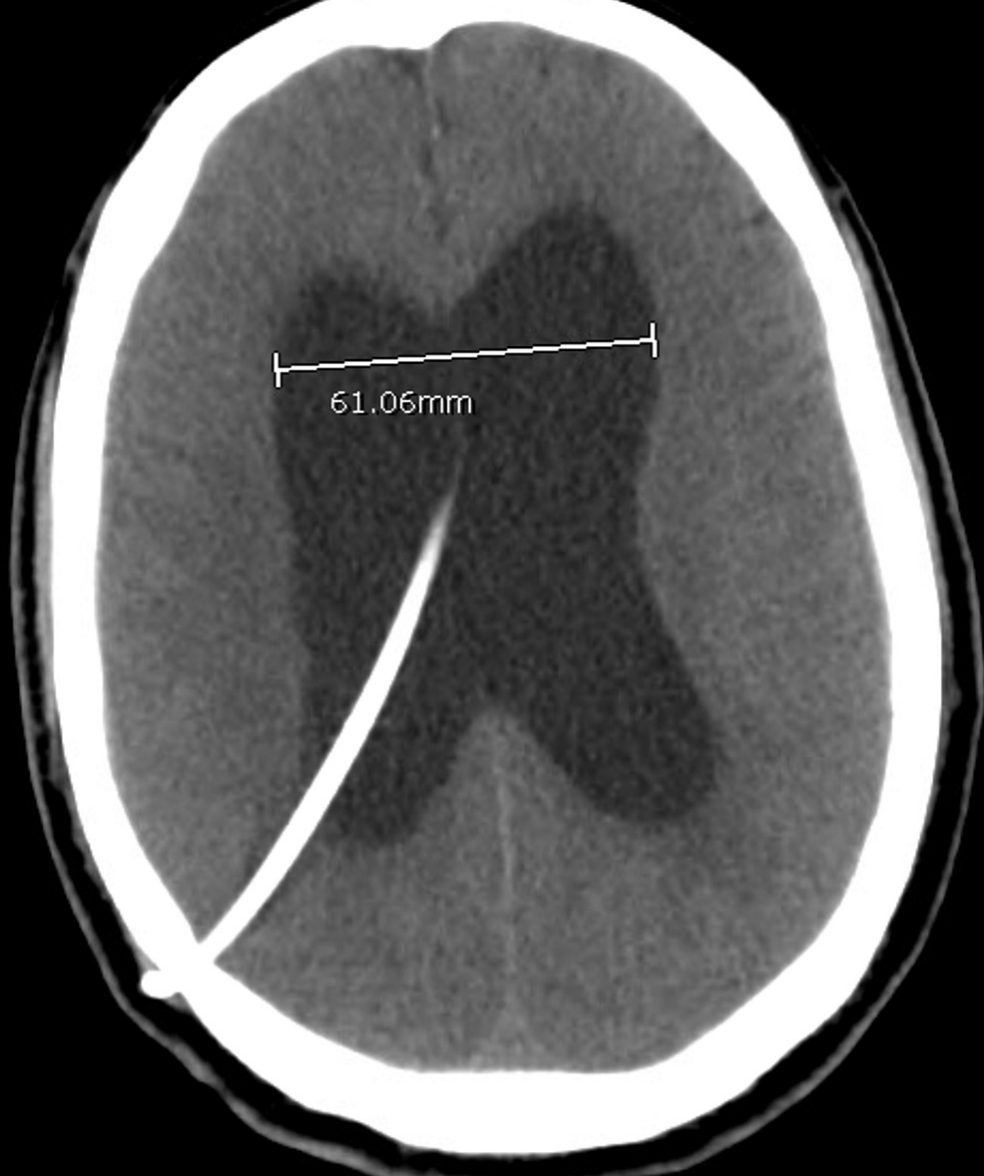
CT brain after the insertion of the VP shunt re-demonstrating ventriculomegaly CT: computed tomography; VP: ventriculoperitoneal
